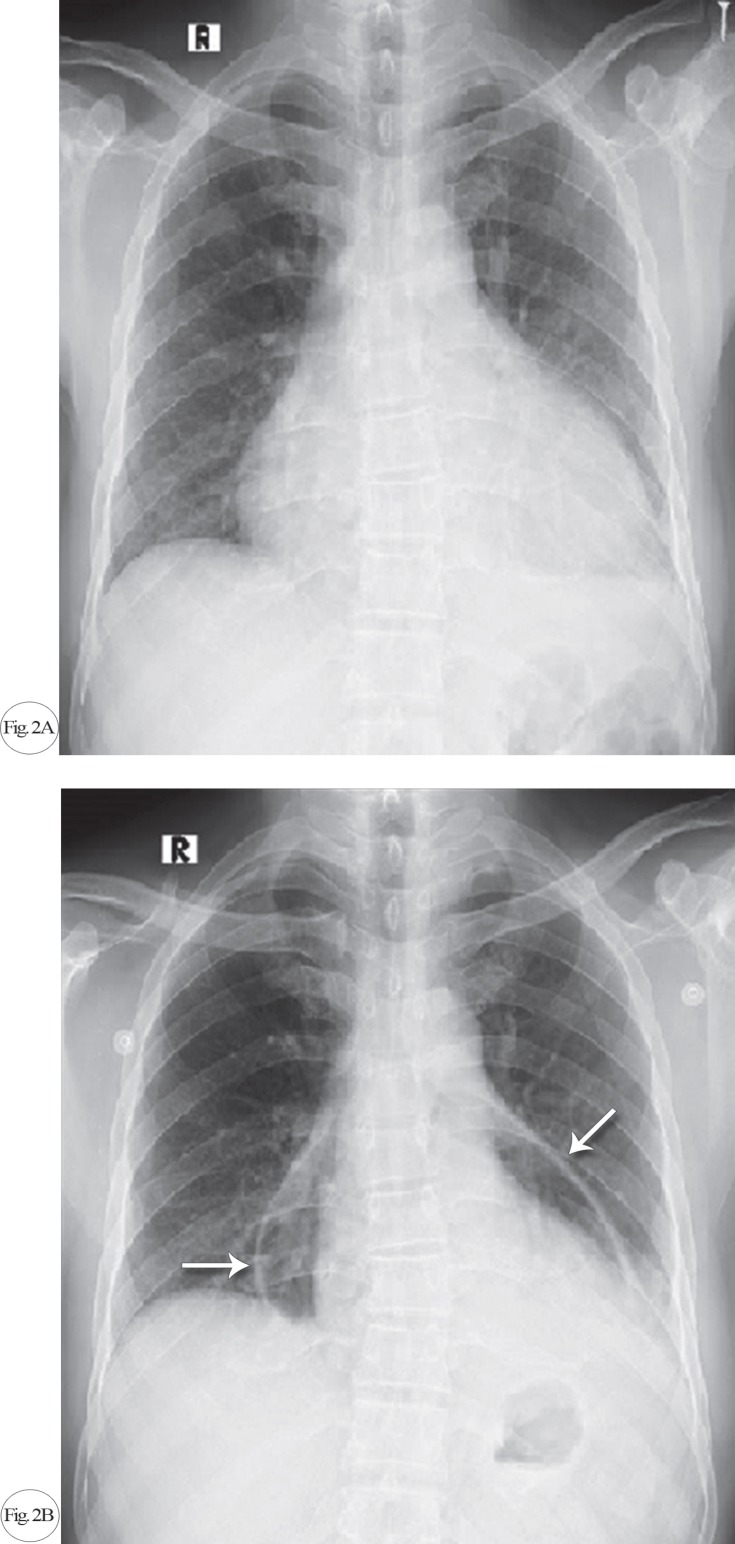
During his stay in the CCU, the patient developed atrial fibrillation, which was pharmacologically cardioverted after heparinization with enoxaparin and 300 mg of amiodarone administered intravenously stat in 100 mL of normal saline infused over 30 minutes. The sinus rhythm was maintained, and a maintenance dose of 600 mg of amiodarone was infused intravenously over 24 hours. After 48 hours of strict observation and vital monitoring, he was transferred to his room. Repeat chest X-ray was done after 72 hours, and it showed a reduction in the pneumopericardium. He made an uneventful recovery and was discharged home on empiric anti-tuberculosis therapy. A follow-up review after 2 weeks showed the complete resolution of the pneumopericardium with no pericardial recollection.
